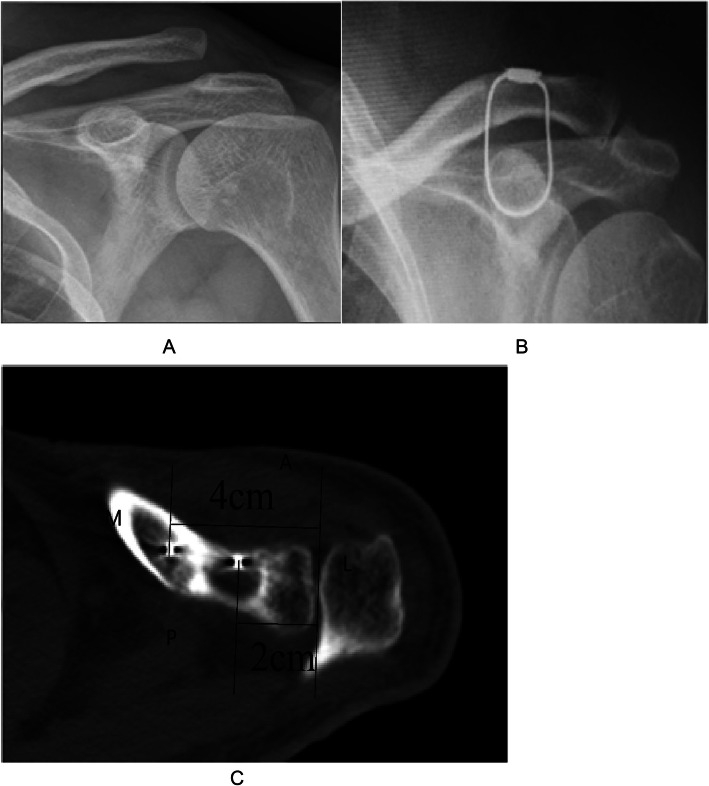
(a) Preoperative X-ray of the shoulder; (b, c) Postoperative X-ray and CT of the shoulder at the final follow-up 12 months after surgery
